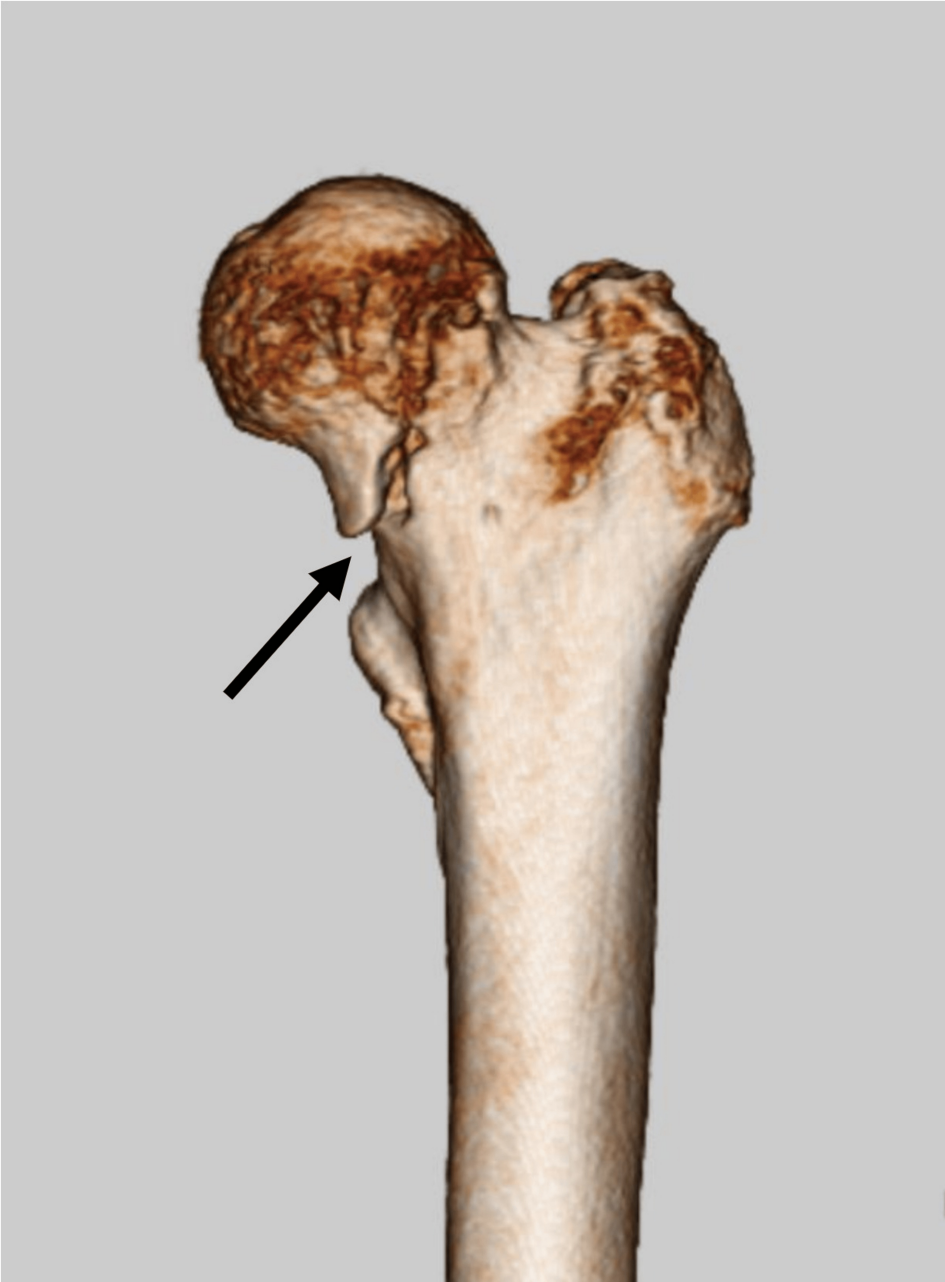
Preoperative three-dimensional computed tomography of left femur
